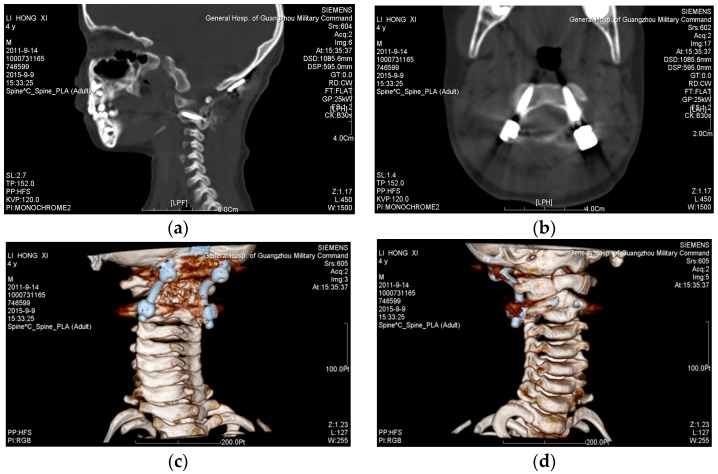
The surgical results observed through the CT reverse engineering method. (a) Sagittal view; (b) transverse view; (c) 3D orientation 1; and (d) 3D orientation 2.
